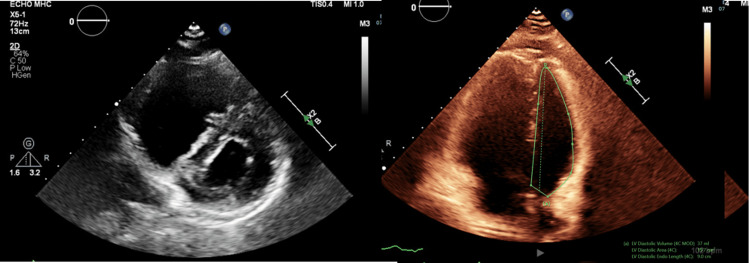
Echocardiographic findings indicate right ventricular dilatation and pulmonary hypertension
